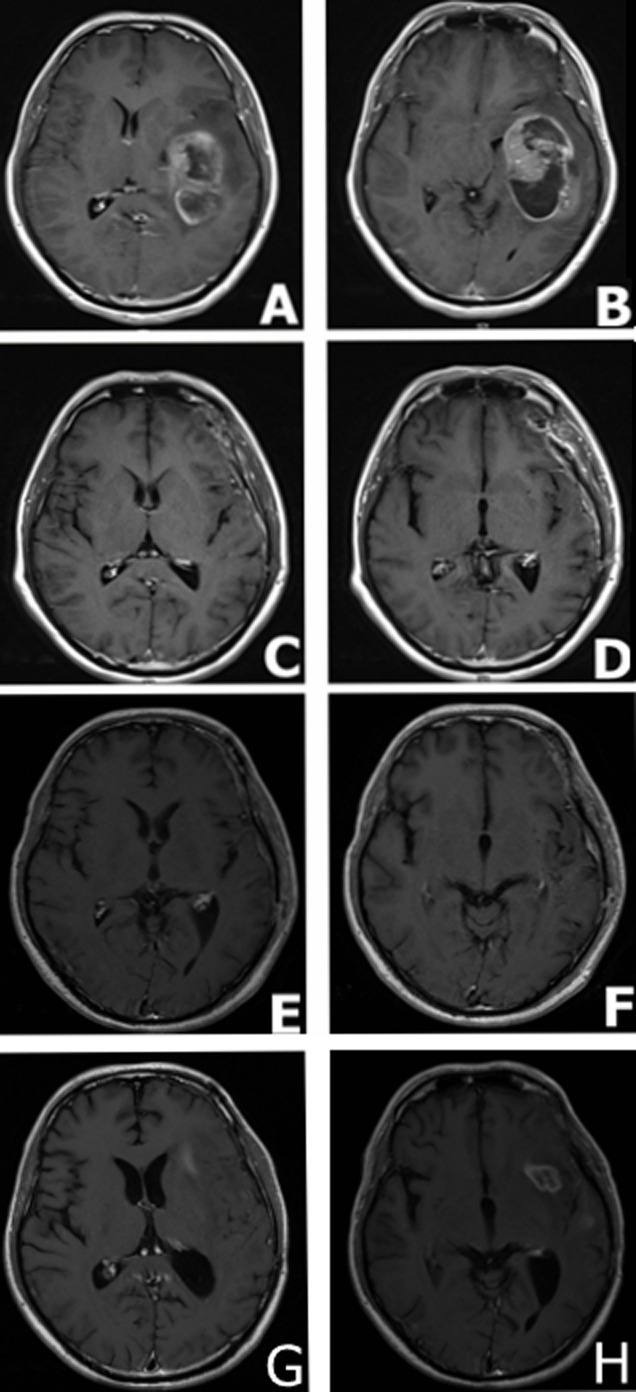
MRI findings in a male patient presented with glioblastoma multiforme A. and B. MRI scans at the disease onset demonstrating a large mass in the left temporal parietal area with marked surrounding edema and a shift of the midline structures to the left side. C. and D. MRI scans captured at one month following surgical resection; MRI demonstrating gross total resection. E. and F. MRI scans at 5 months following surgical resection, standard radiation and concomitant chemotherapy demonstrated the absence of tumor relapse. G. and H. MRI scans at 7 months following surgical resection: MRI demonstrated tumor relapse.
